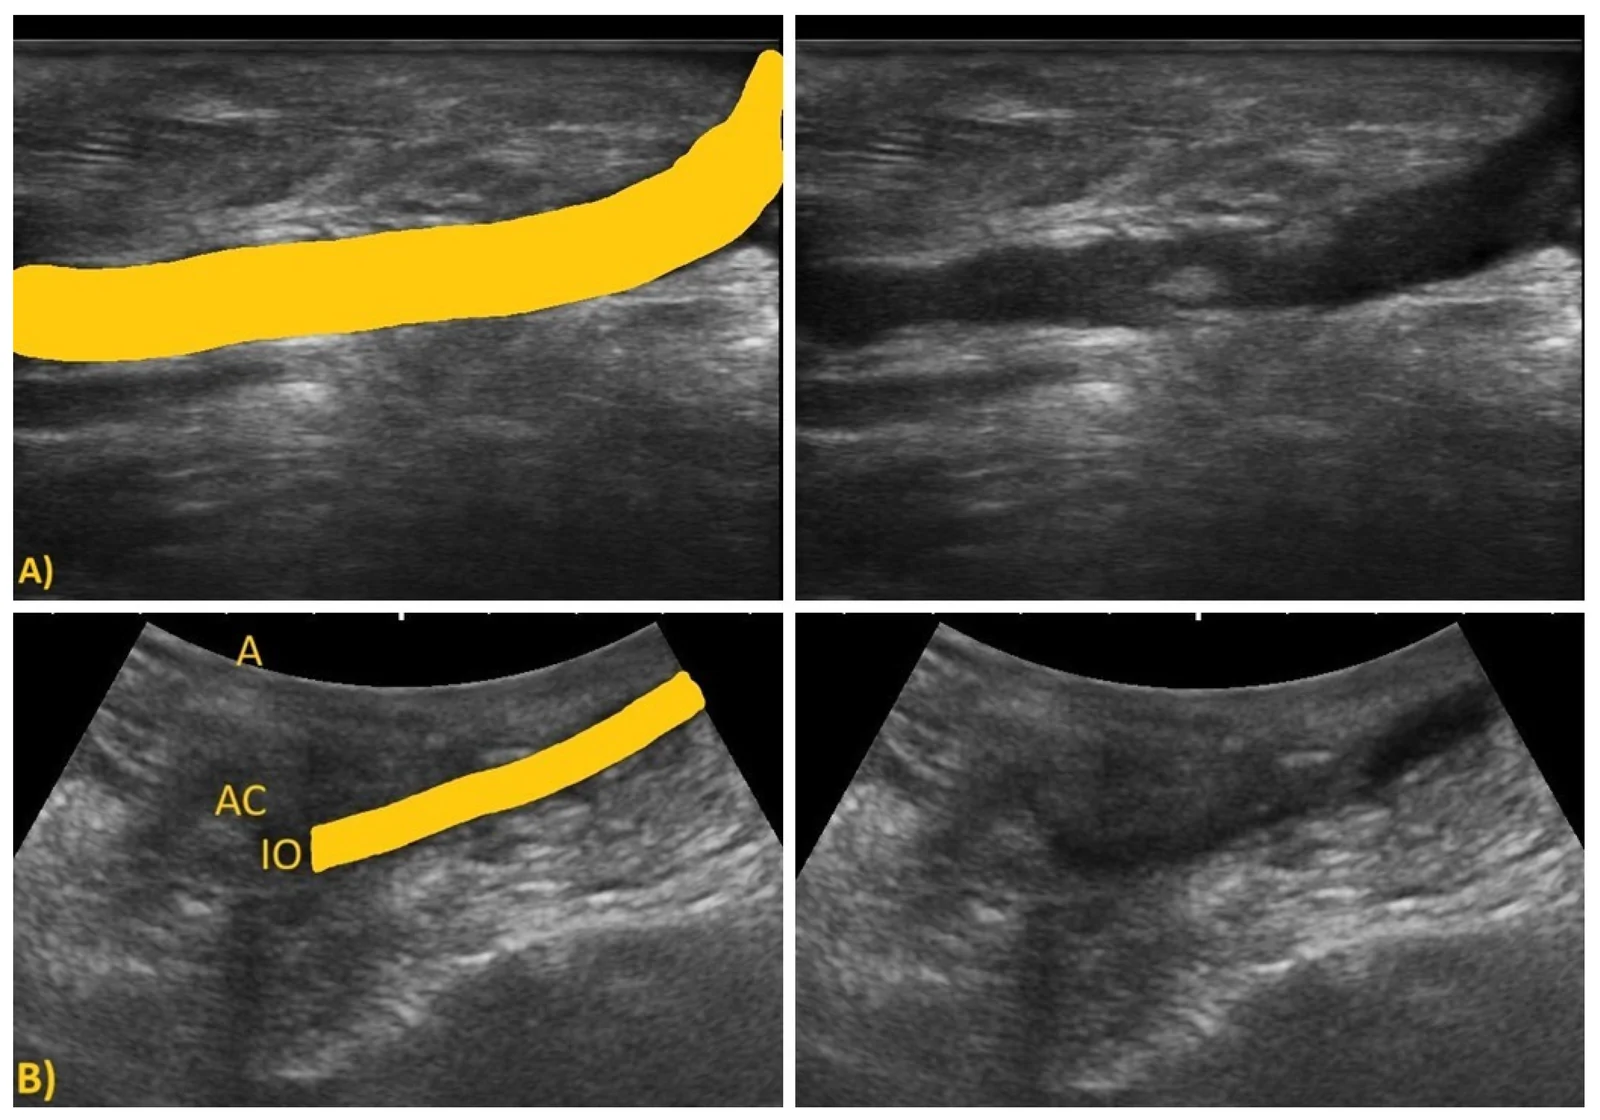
Ultrasound images: (A) Images obtained using a superficial linear probe (7.5 MHz) show a long, low-type fistulous tract, making it difficult to visualize the external opening (EO) and internal opening (IO) on the same scan. (B) Images obtained using a deep curved probe (3.5 MHz) show a 7 cm long fistulous tract with an obvious internal opening (IO) into the anal canal (AC). However, the external opening was difficult to visualize on the same scan due to the length of the fistula. The left images show the fistulous tract (thick yellow line) and the IO. The right images are the corresponding unannotated scans. A: Anal verge.
